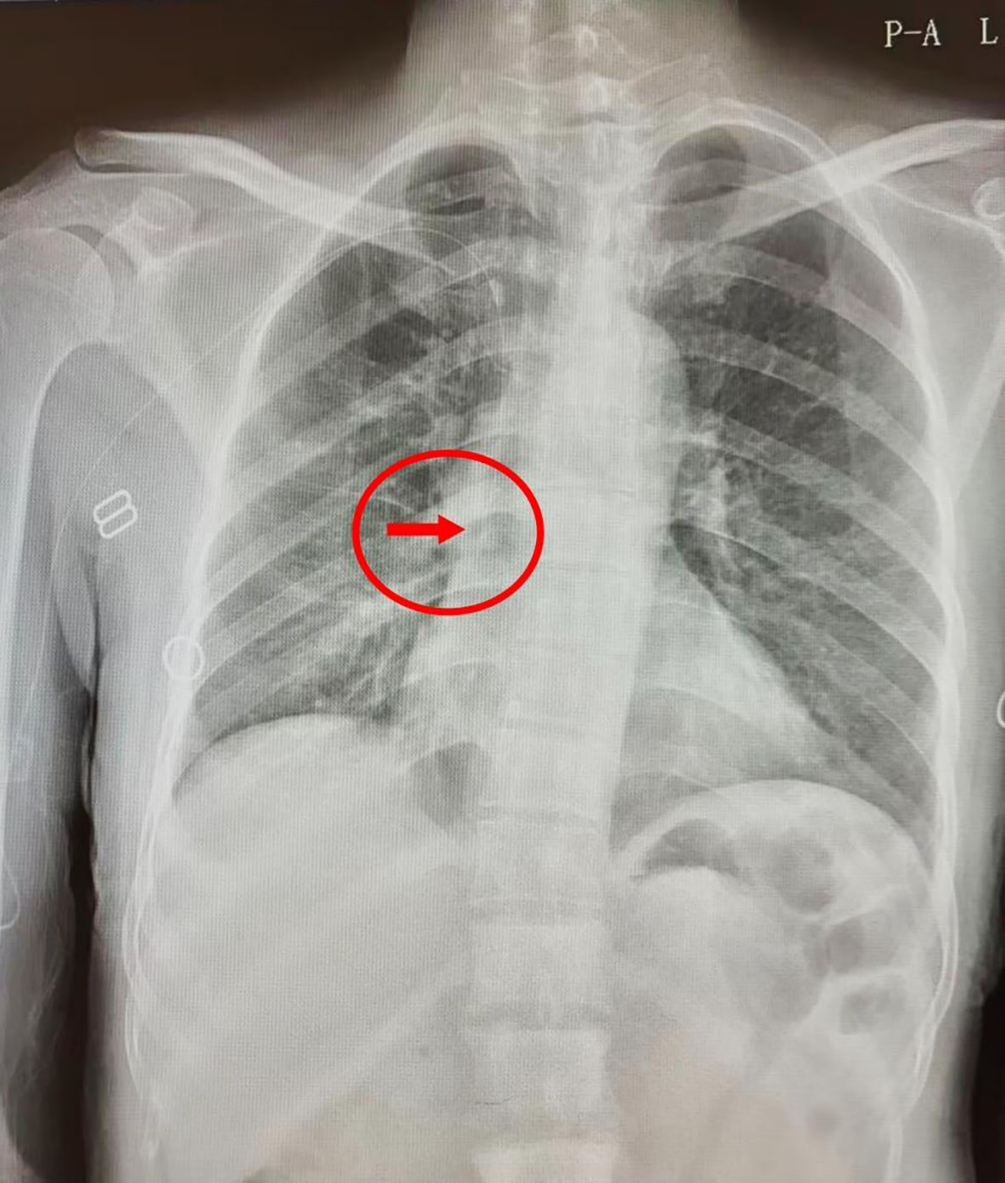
A post-procedural chest X-ray confirmed that the PICC tip was located at the lower edge of the T6/7 vertebra.
